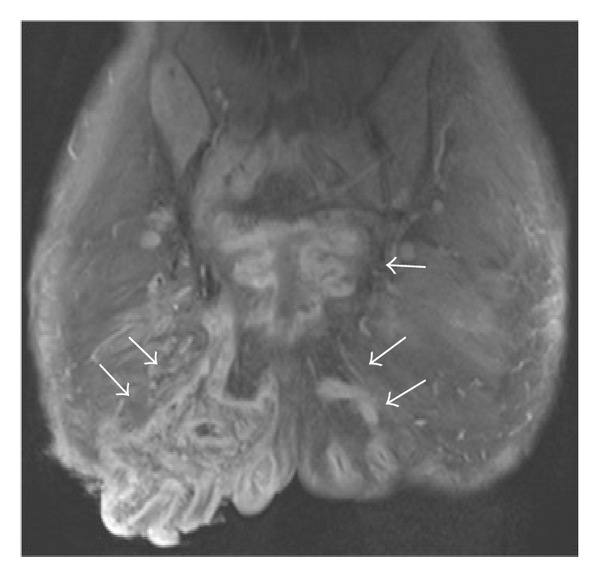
Coronal FSE T1-weighted fat-suppressed post-IV Magnevist 10 mls. Note: rim-enhancement of these collections post-IV contrast (white arrows), consistent with abscesses.
